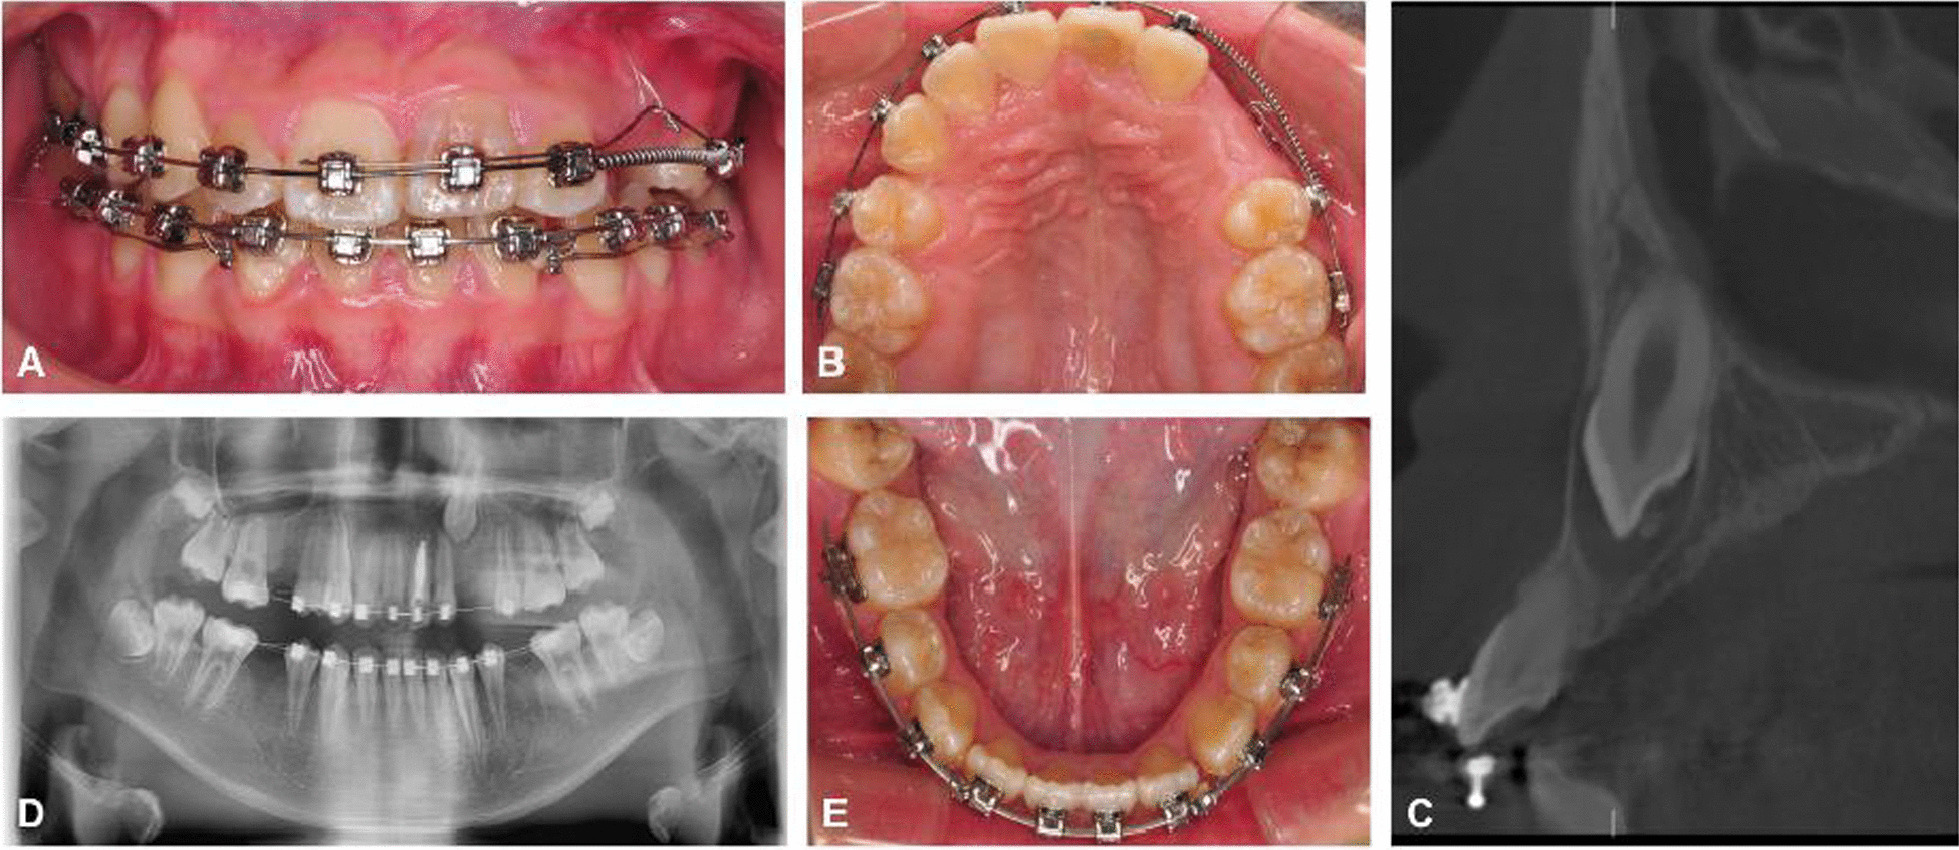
Evaluation of the impacted teeth: clinical photographs (A, B, E), CT scan (C) and orthopantomogram (D)
